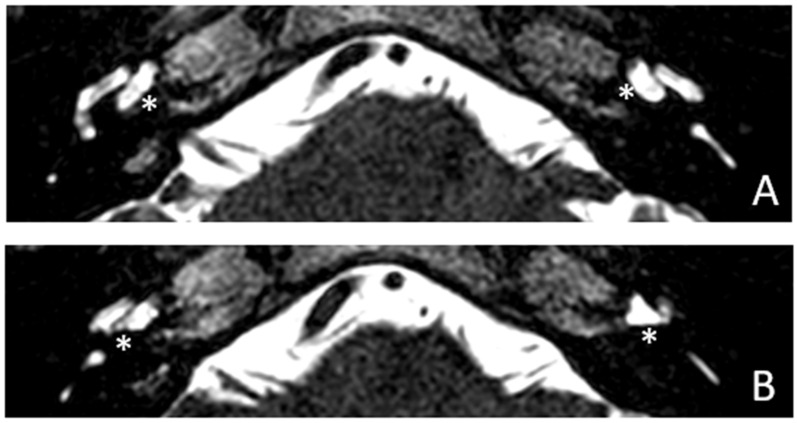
MR images of patient #3 with otosclerosis and SSC dehiscence on the left side. MR cisternography: (A,B), axial plane. Note the fluid-signal in the newly formed cavity (white asterisk).
